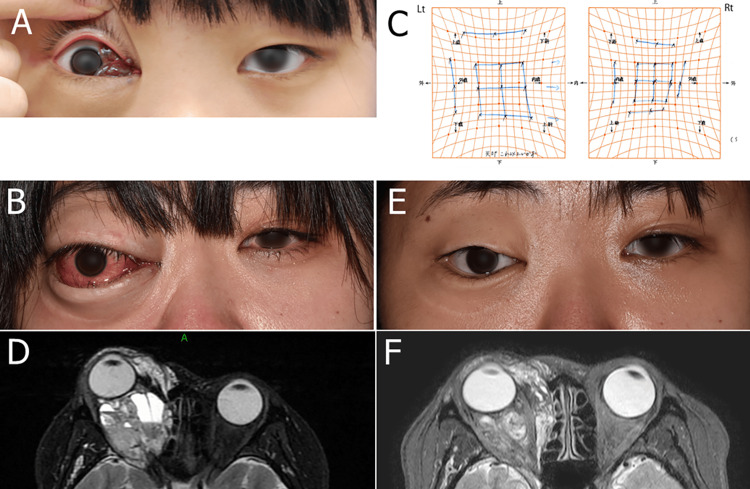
Case 1 (A) A photograph of the right eye at the age of 13 shows a blue-purple cystic lesion in the medial canthal region. (B) The patient before treatment demonstrates distinct proptosis and chemosis. (C) The Hess chart before treatment indicates a marked right eye adduction disorder. (D) A T2-weighted MRI image taken before treatment reveals a high-signal lesion with niveau formation in the posterior eyelid and muscle cone. Volumetry showed that the lesion volume was 43.9 cm³. (E) The patient, six months after starting sirolimus, shows remarkable improvement in proptosis and chemosis. (F) A T2-weighted MRI taken six months after starting sirolimus shows that the lesion volume was reduced to 31.4 cm³, indicating a reduction rate of 28.5% compared to the volume before treatment. MRI: magnetic resonance imaging
